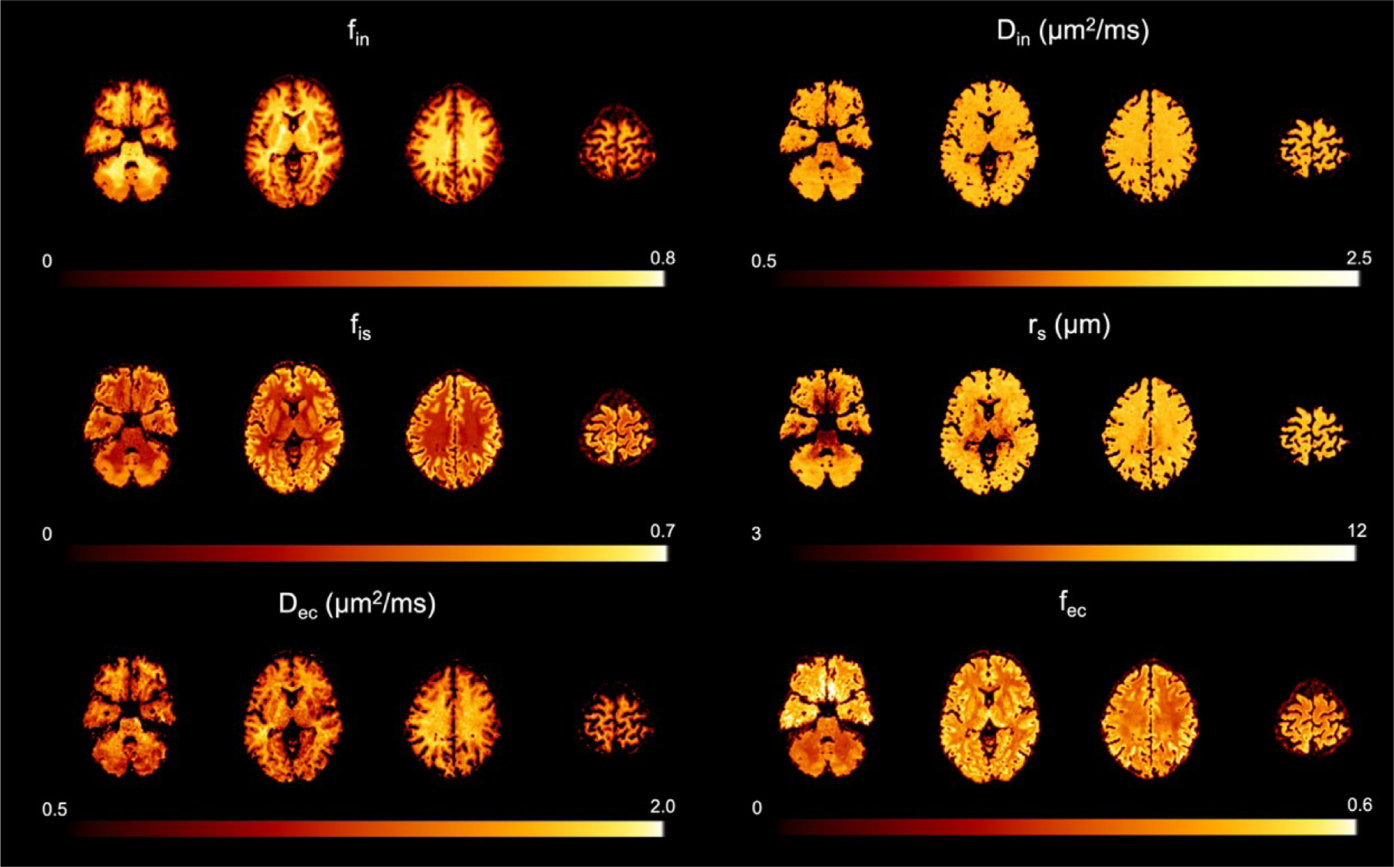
An example of the parametric maps of the proposed compartment model for brain microstructure, obtained by fitting Eq. (10) to the normalised direction-averaged DW-MRI data from a representative subject.
